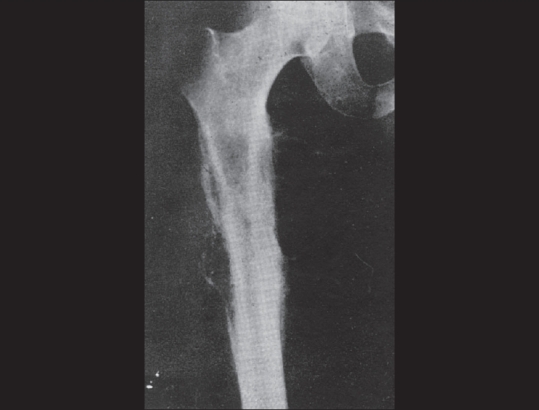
Ewing's sarcoma of the proximal femur. The plain radiograph shows a heterogeneous appearance with a bizarre pattern of periosteal and subperiosteal reaction
